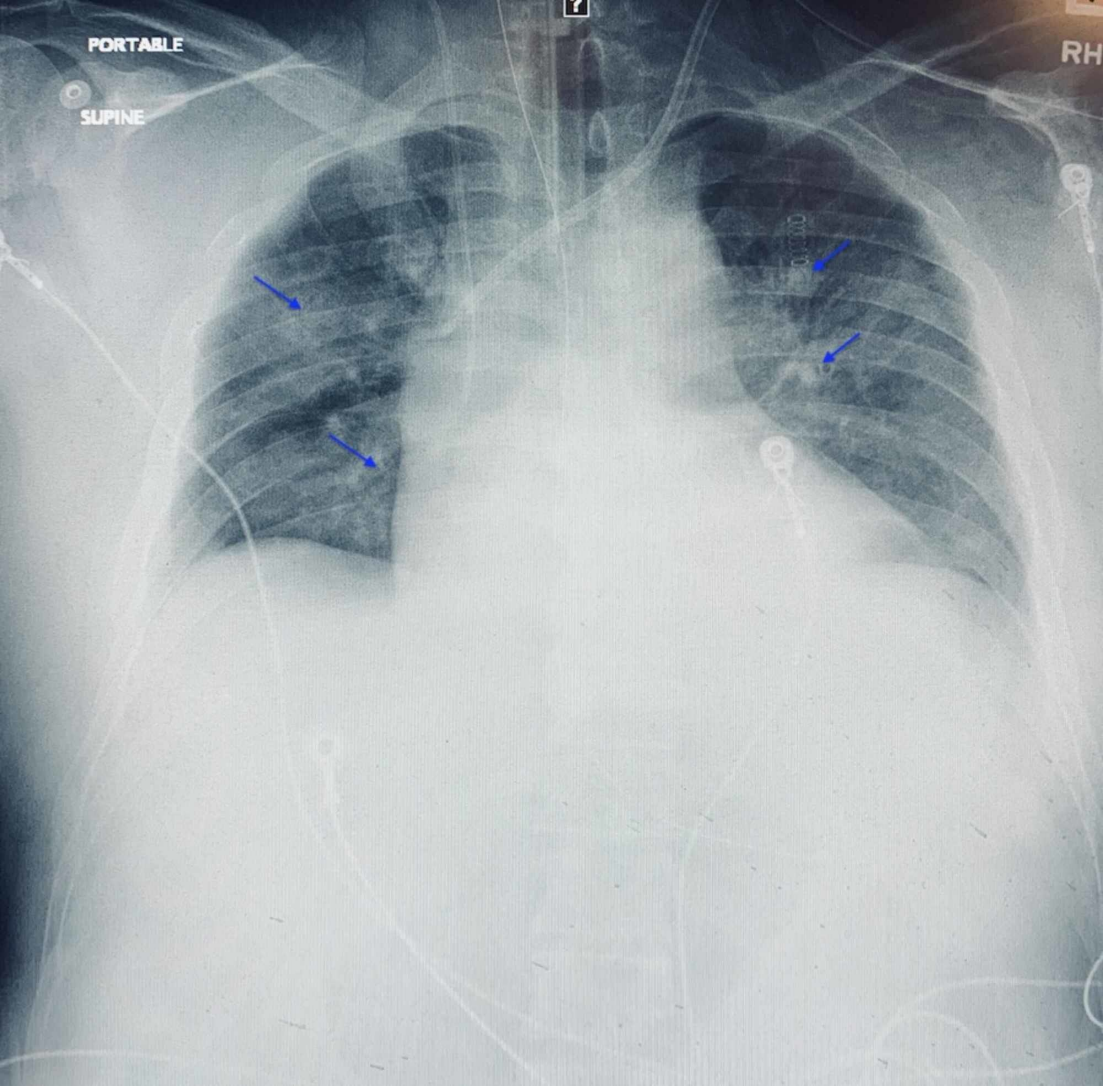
Chest radiograph demonstrating mild bilateral perihilar airspace opacities (arrows)
